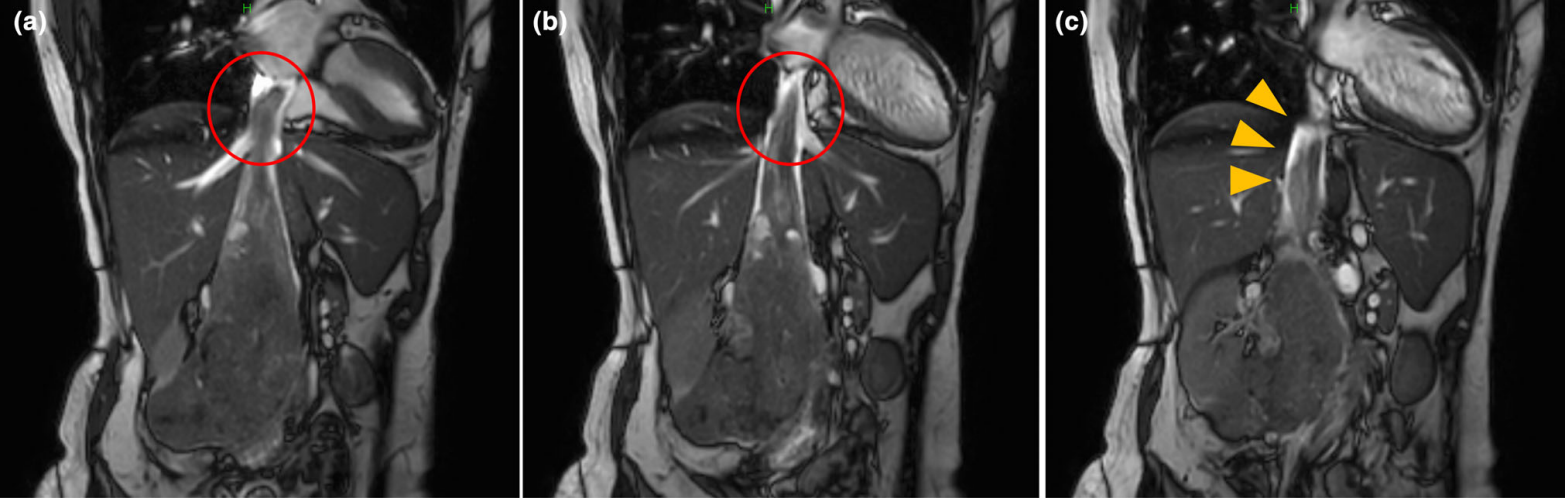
Coronal views of cine MRI before surgery showing the (a) tumor apex, (b) superior portion of the IVC, and (c) inferior portion of the IVC. The red circle indicates the apex of the tumor. Blood flow is seen between the IVC and the tumor (yellow arrows). The images confirm mobility of the top margin of the tumor within the right atrium and indicate the absence of adhesions between the tumor and the IVC wall.
